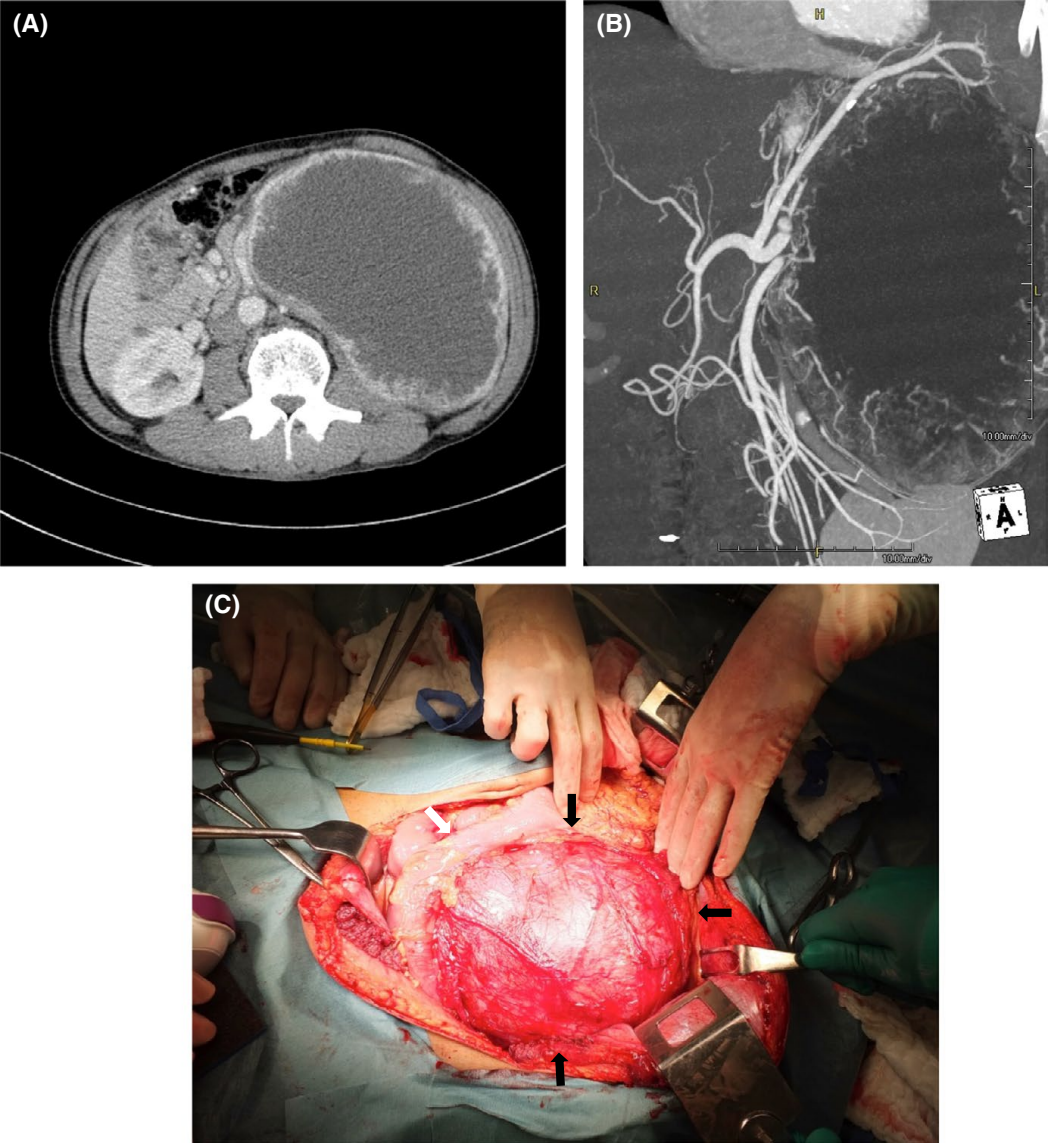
Case 1: A and B, preoperative abdominal computed tomography (CT) showing a giant., hypervascular left adrenal tumor measuring 21 × 19 × 12 cm. C, intraoperative photograph (laparotomy) showing a large pheochromocytoma (black arrows) at the caudal end of the transverse colon (white arrow)
